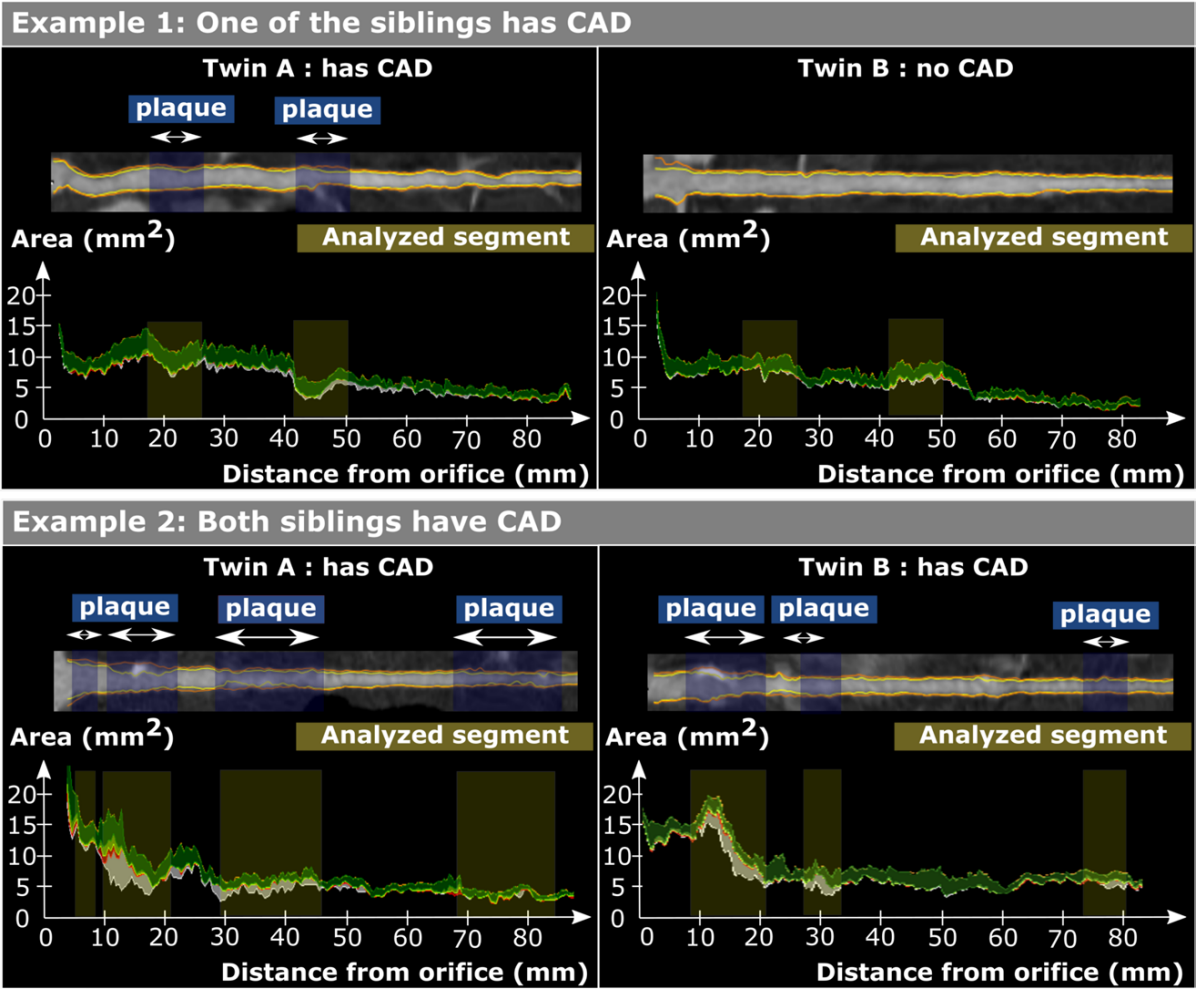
Demonstration of the plaque analysis method. Stretched multiplanar reconstruction of the left anterior descending (LAD) artery and quantification graph of the LAD. Yellow shaded regions correspond to analyzed segments and blue shaded regions correspond to plaques. Components of coronary plaque are shown as follows: lipid-rich (low-computed tomographic attenuation) plaque components are shown in red; fibro-fatty tissue is shown in light green; fibrous tissue is shown in dark green; calcium is shown in white. Example 1: One of the siblings had plaques; plaques were analyzed, and for the other sibling, segments in the same location and same length were analyzed. Example 2: Both siblings had plaques; all the segments were analyzed which included any plaque in either of the siblings. In case neither of the siblings had coronary artery disease (CAD), a plaque volume of 0-0 was used.
